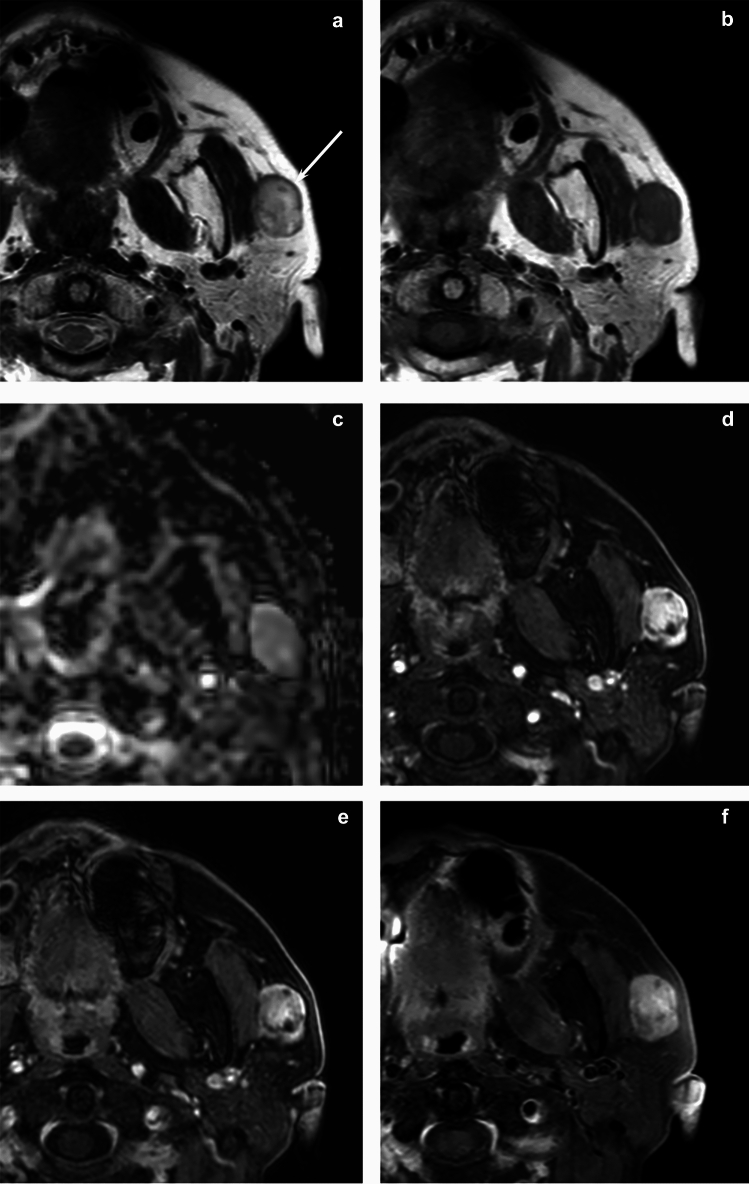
A 60-year-old woman with myoepithelioma of the left parotid gland. a T2-weighted image shows a heterogeneously mild hyperintense lesion (arrow). b T1-weighted image shows heterogeneously hypointense relative to the spinal cord. c ADC map shows high ADC value (1.68 × 10^−3^ mm^2^/s). d Fat-suppressed dynamic contrast-enhanced T1-weighted image 30 s after intravenous administration shows heterogeneously strong enhancement. e. Fat-suppressed dynamic contrast-enhanced T1-weighted image 180 s after intravenous administration shows mild washout. f Fat-suppressed contrast-enhanced T1-weighted image shows moderate enhancement with focal unenhanced areas
